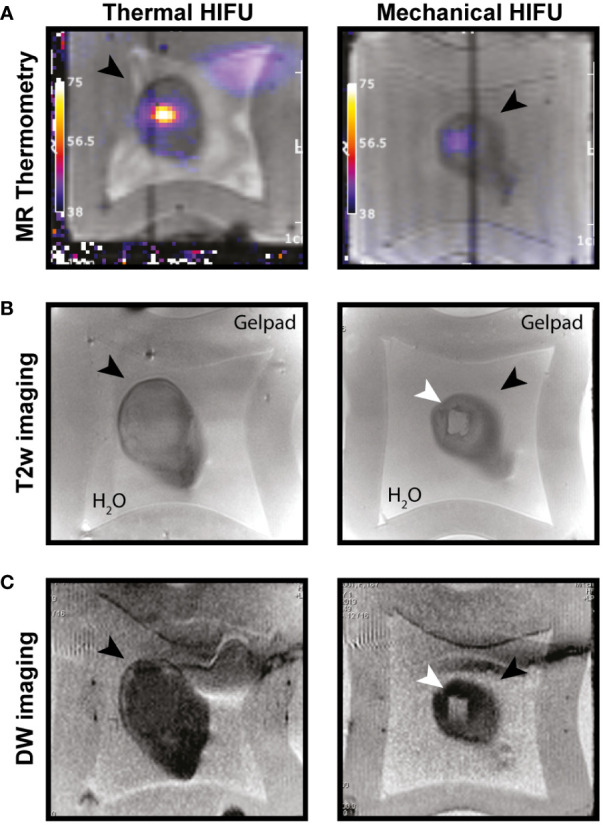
MR imaging of T-HIFU and M-HIFU ablation. Coronal MR images of EG7 tumors during (A) and immediately after (B, C) thermal (T-) and mechanical (M-)HIFU ablation (tumor, depicted by black arrowhead, is surrounded by water and a gelpad). (A) T1 weighted image with temperature map overlay during T-HIFU (left) or M-HIFU (right) ablation. Temperature map shows high temperature increase during T-HIFU ablation. (B) Coronal T2 weighted MR images and (C) DW images recorded immediately after T-HIFU (left) or M-HIFU (right) treatment. Both T2 weighted and DW images reveal a hyper-intense lesion after M-HIFU treatment (indicated with white arrowhead). DW: diffusion weighted; HIFU, high intensity focused ultrasound; MR, magnetic resonance; T2w, T2 weighted.
